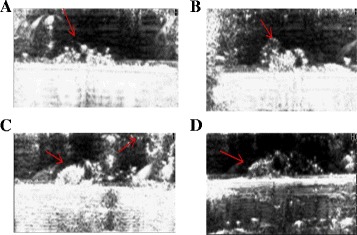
Typical plaques in the rabbit carotid as imaged with ultrasound at 12 MHz. In three of the cases, the reduction occurs in one side of the rabbit carotid artery (A, B, D). In one case, the plaque appears on both sides of the artery (C). Plaques are indicated with arrows.
